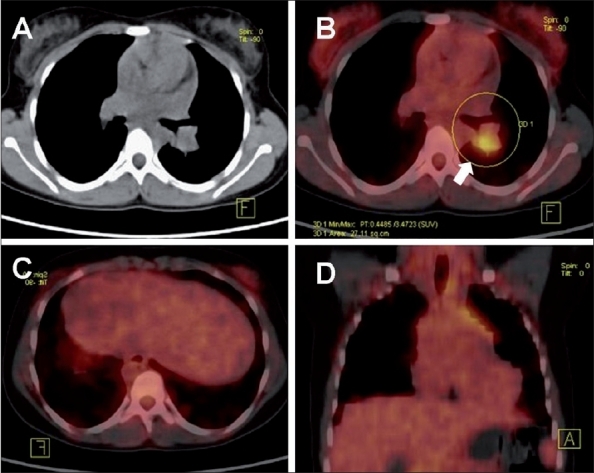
Repeat axial CT (A), PET/CT (B) images of the thorax, and axial (C) and coronal (D) PET/CT abdomen images of the same patient described in Figure 5 show complete resolution of the liver lesion and a considerable reduction in the size of the left hilar mass and the intensity of the FDG uptake (arrow) (SUV_max_ 3.4). PET was useful in assessing response to therapy
